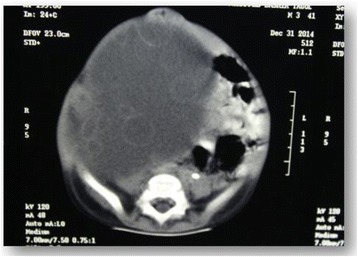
CT abdomen shows a *large* cystic mass arising from the right kidney
